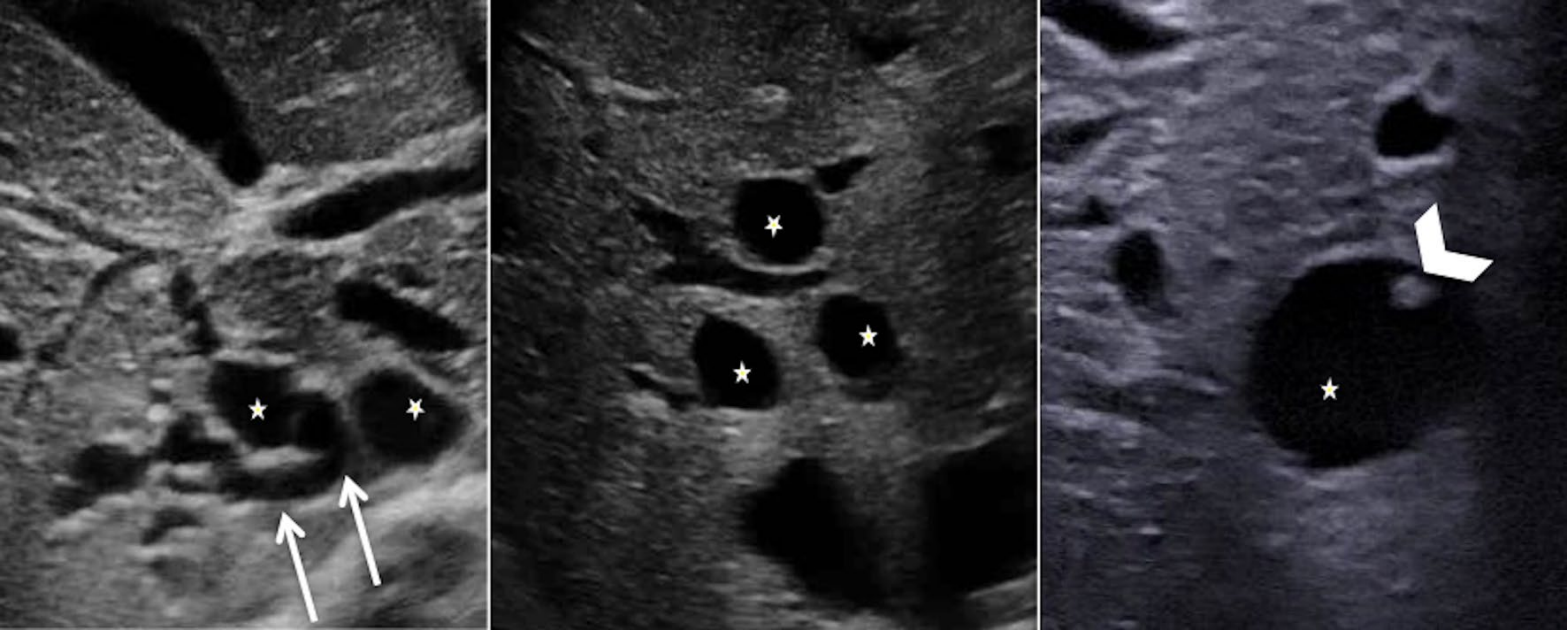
Caroli syndrome in a 7-year-old female, at ultrasonography. Several round anechoic cysts (stars) with thin wall, increased through-transmission, connected to the dilated fusiform biliary tract (arrows), some of which show the highly specific “central dot sign” (arrowhead)
